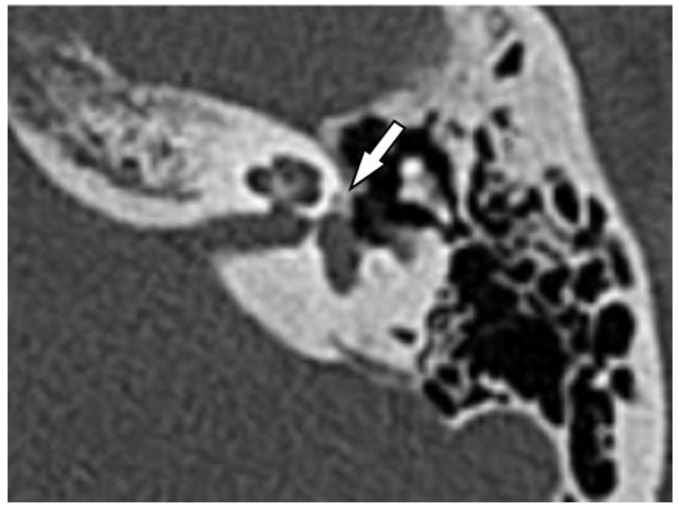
Non-contrast high-resolution temporal bone computed tomography (HRCT) image in the axial plane showing a temporal bone with surgically confirmed otosclerosis. The white arrow indicates a fenestral otosclerotic focus that was detected in the HRCT images by the subspecialist neuroradiologist in the second reading but missed by the general radiologist in the clinical routine reading.
